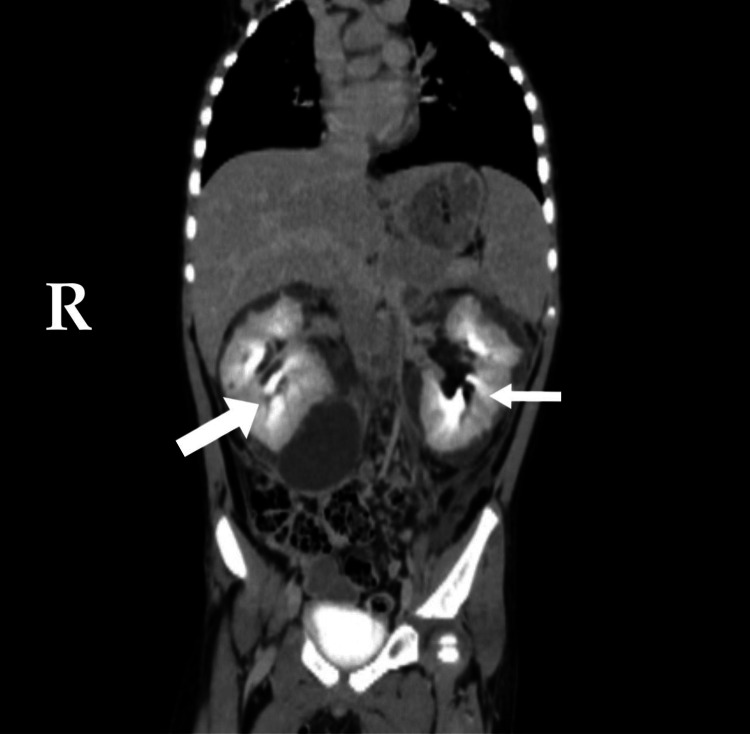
Contrast-enhanced CT of the abdomen (coronal view). There is compression of the renal parenchyma bilaterally (white arrows)
